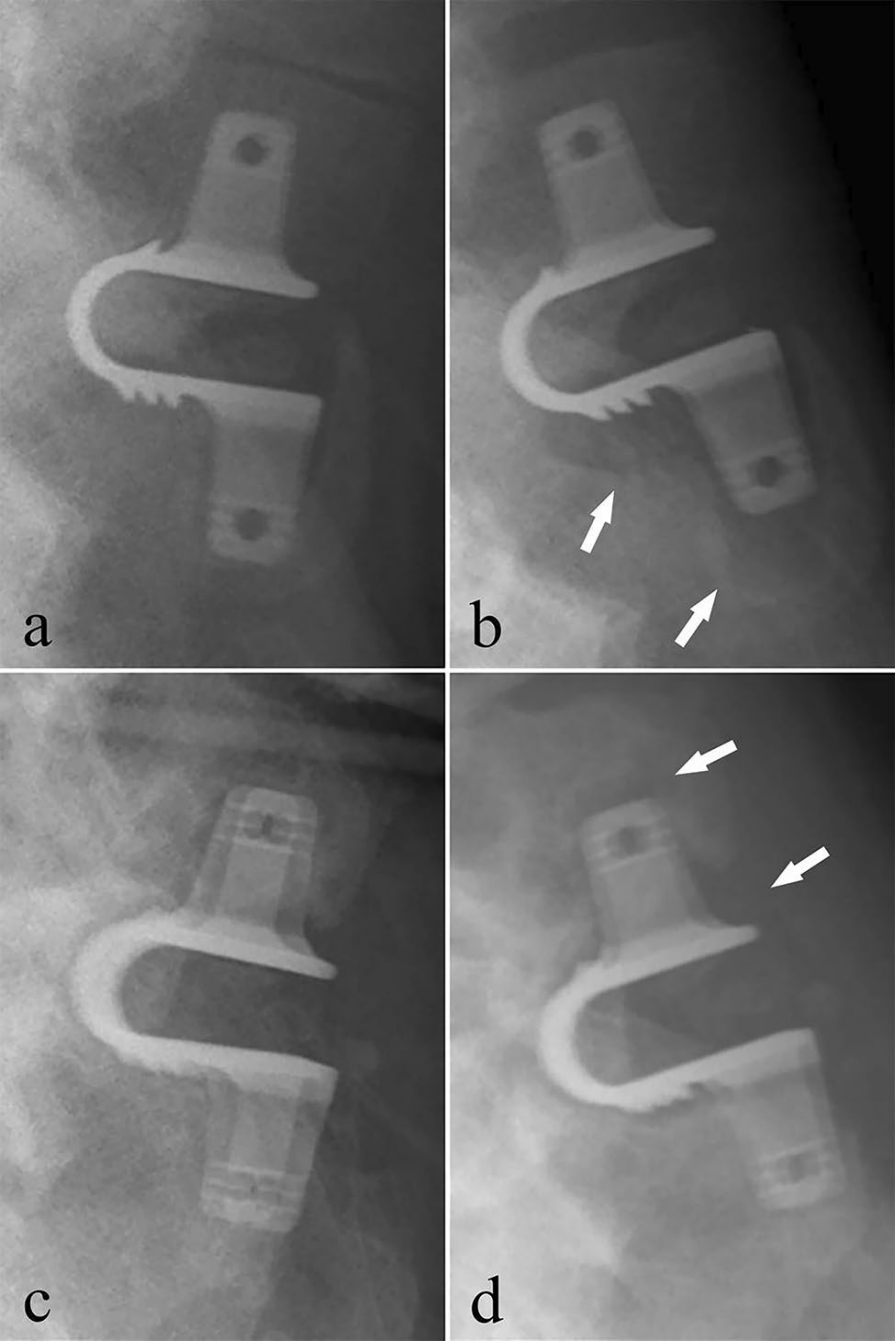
Radiographs of two patients, lateral and over-flexion views. Arrows point out the areas of Coflex loosening in over-flexion position
